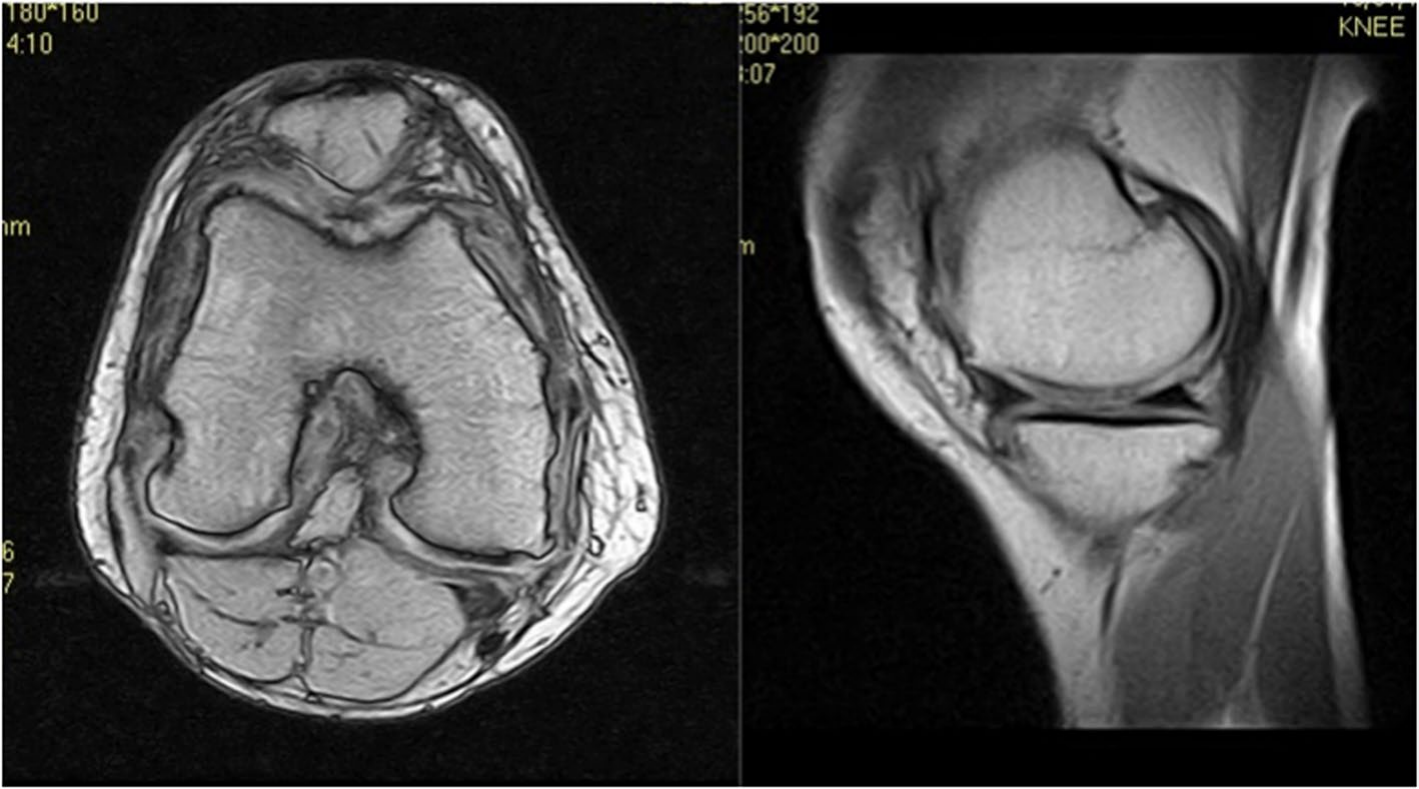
Patient MRI with a grade 4 cartilage lesion in the trochlea, patella and medial femoral condyle. 395 × 219 mm.^2^ (144 × 144 DPI)
